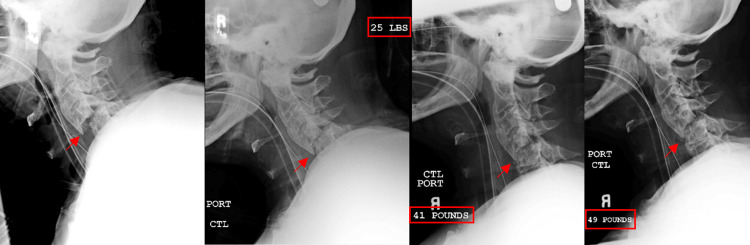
Sequential lateral plain film radiographs showing the patient undergoing closed reduction with sequential increase in applied weight. The amount of weight is denoted in each film with a red box. A fracture line is demonstrated in each pane with a red arrow.
